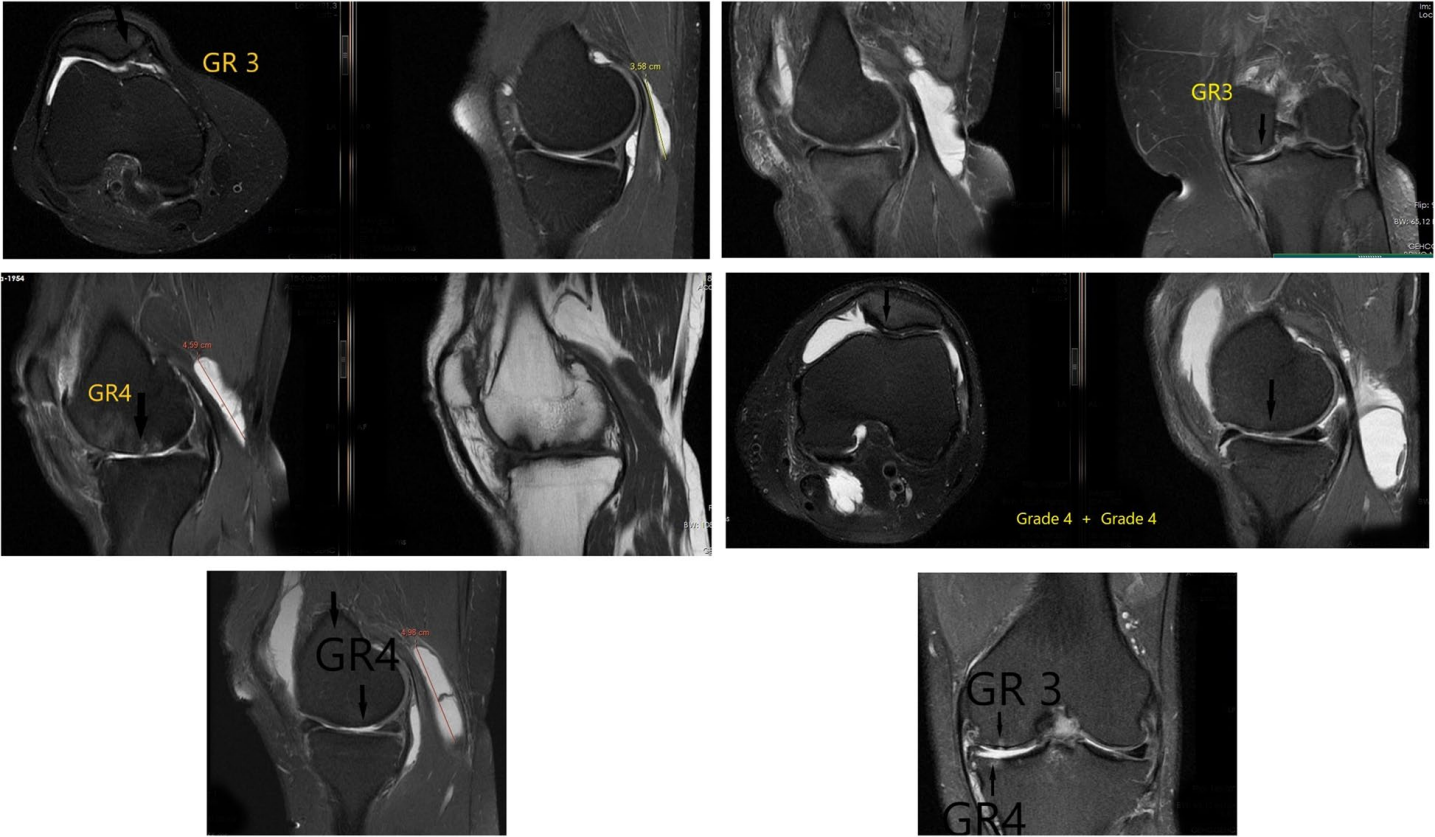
The detailed MRI images of grade 3 and 4 lesions according to the the outerbridge classification
